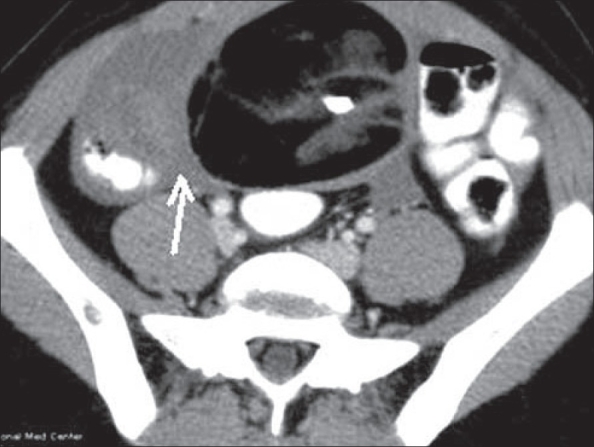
Ovarian dermoid with torsion. Contrast-enhanced CT scan shows a large right ovarian dermoid with high-density peritoneal fluid due to hemorrhage (arrow), strongly suggesting the presence of associated torsion. There were signs of torsion on Doppler USG as well. The appendix was normal
